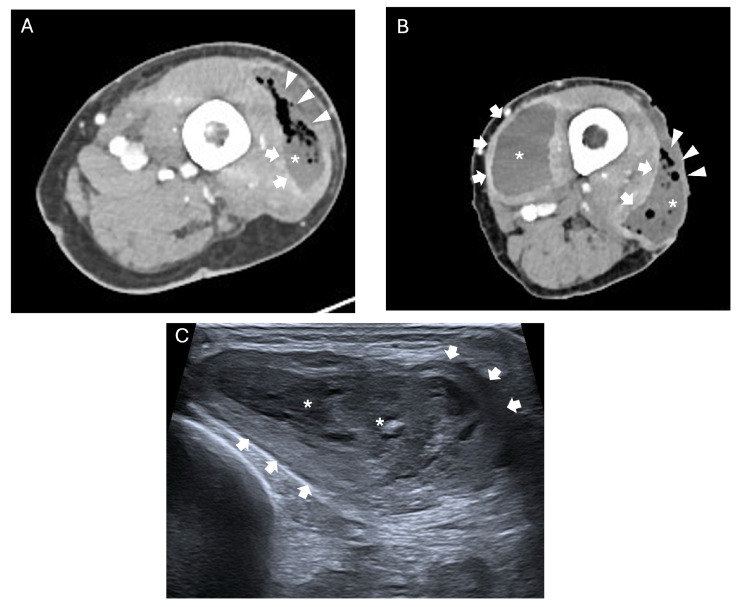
Axial contrast-enhanced CT (A,B) and long-axis ultrasound (C) images of the lower extremity demonstrate the same intramuscular lesion consistent with pyomyositis. On CT it appears as a fluid collection (asterisks) with marked peripheral rim enhancement (arrows) and intralesional gas locules (arrowheads). On ultrasound, a corresponding heterogeneously hypoechoic intramuscular collection (asterisks) with internal debris and a surrounding echogenic capsule (arrows) is observed.
